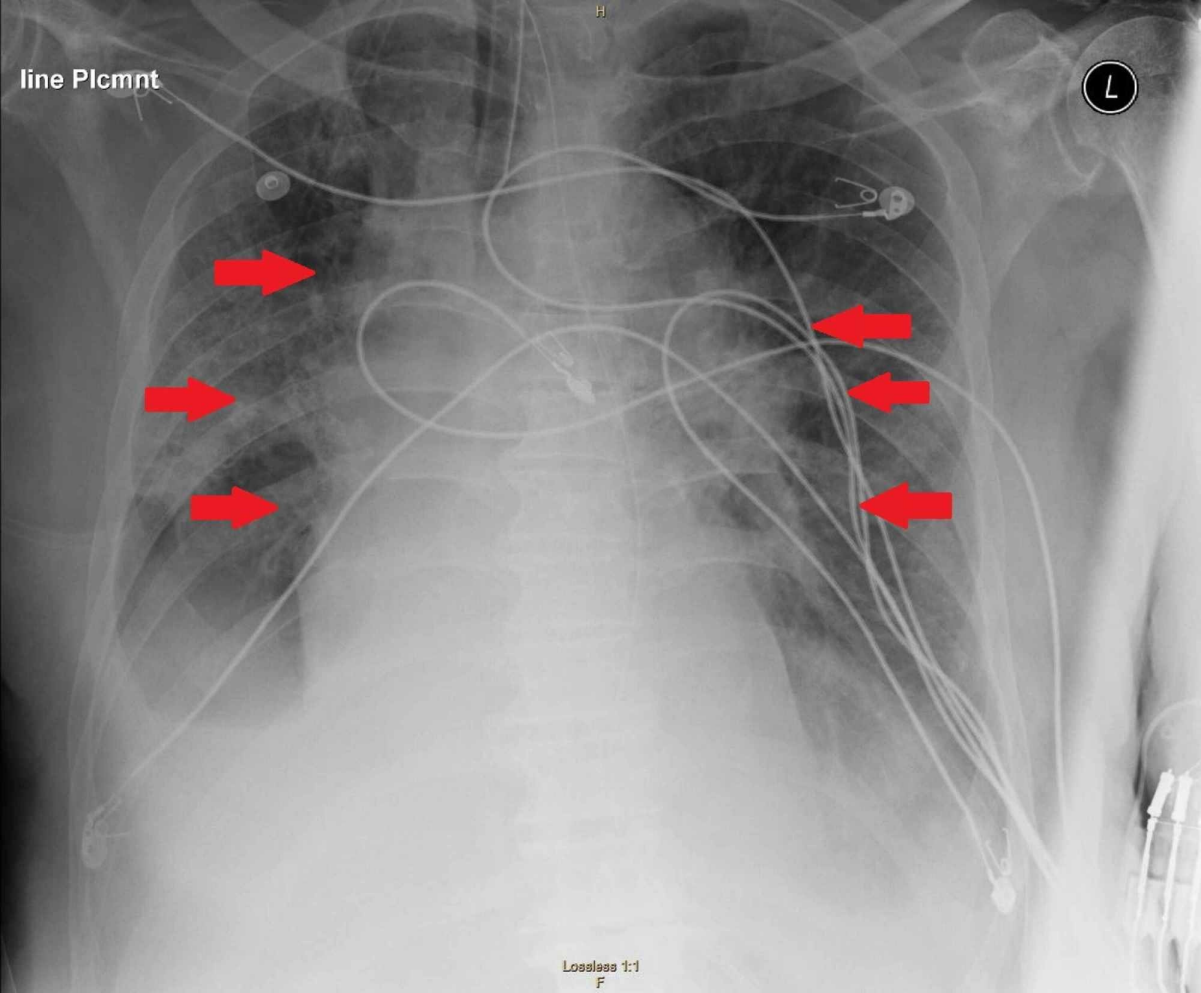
Chest x-ray demonstrating multifocal consolidations concerning for multifocal pneumonia.
